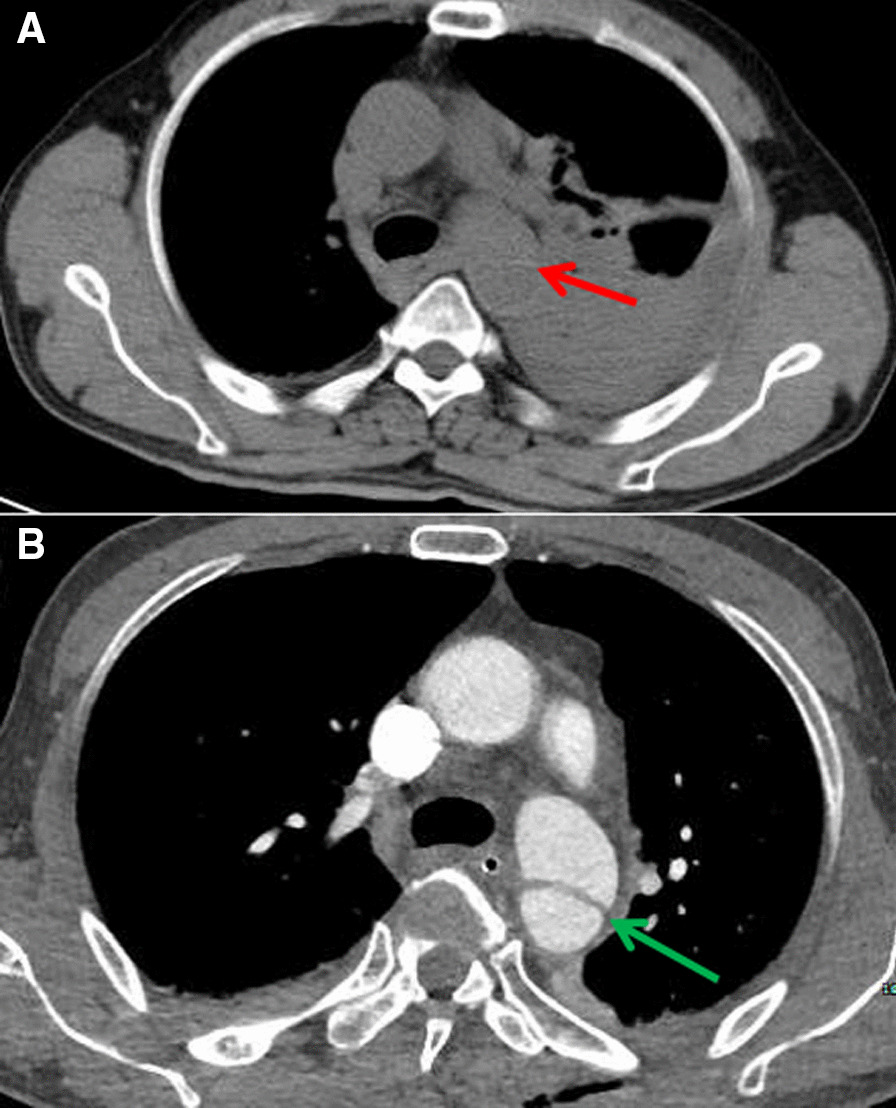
Chest CT imaging scan of Case 2. A The mediastinal space is slightly blurred, with a possible aortic boundary between the true and false lumen (red arrow). B Enhanced chest CT suggests aortic dissection (green arrow)
